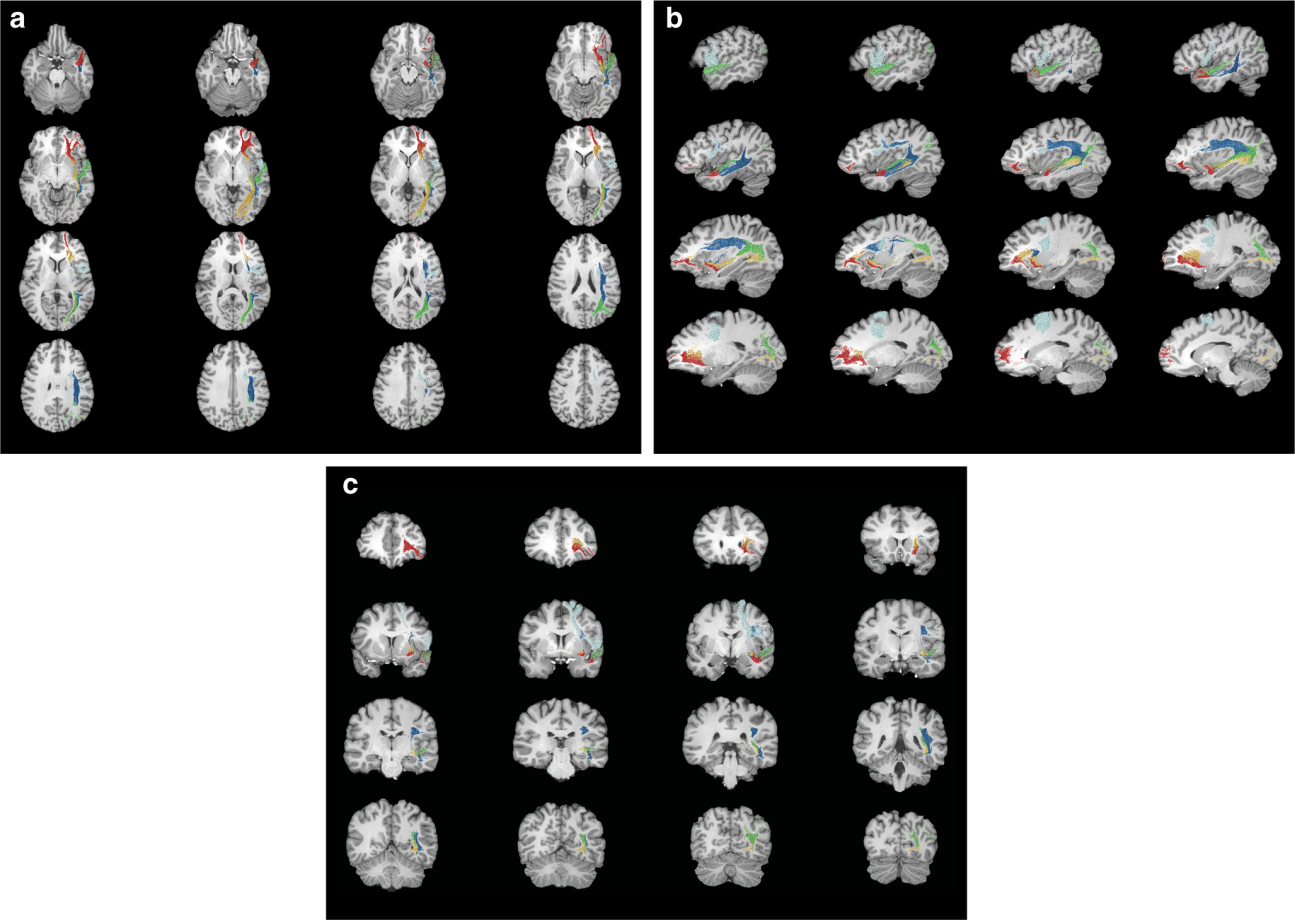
MRI-based lightbox overview with probabilistic fiber tractography results of the five major language fiber bundles. a Axial view. b Sagittal view. c Coronal view
